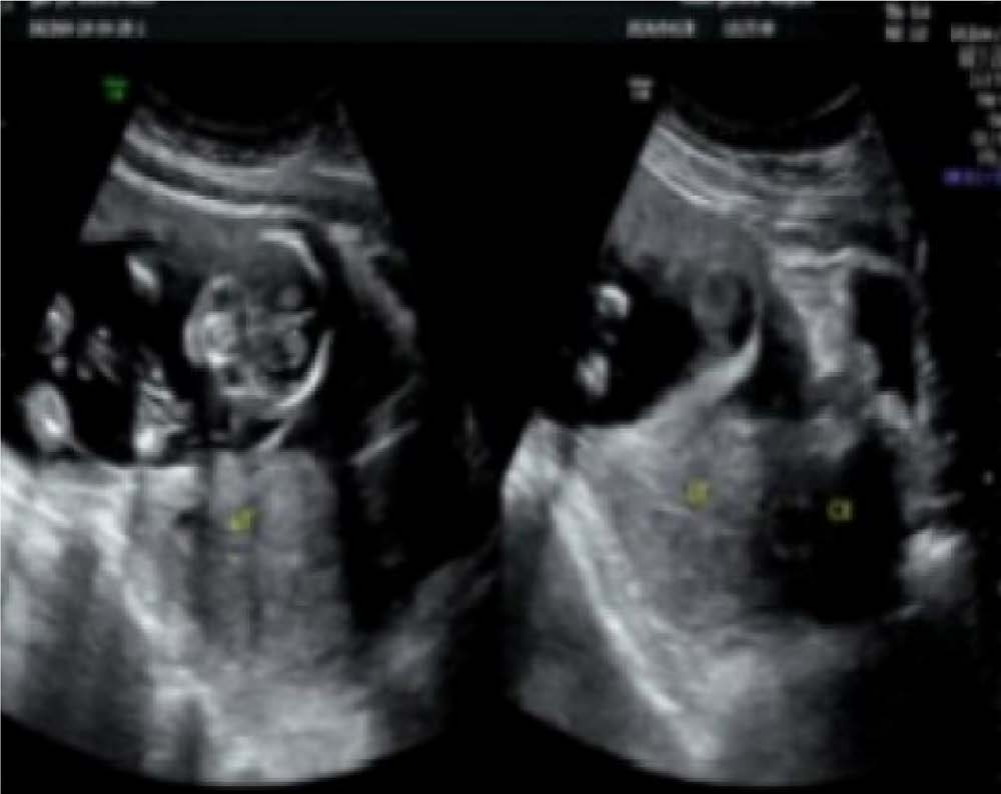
Abdominal pregnancy ultrasound image.
